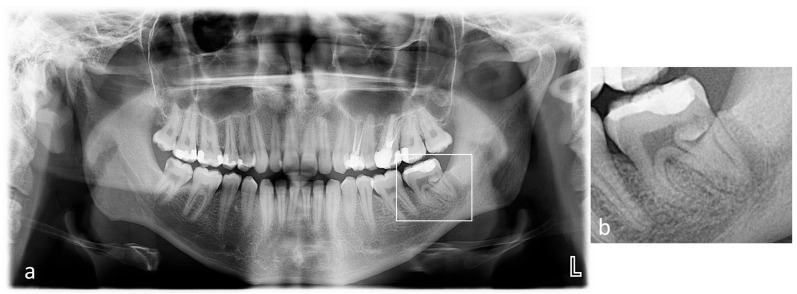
(a) Preoperative Panoramic radiograph; (b) the magnified panel of tooth 3.7.
